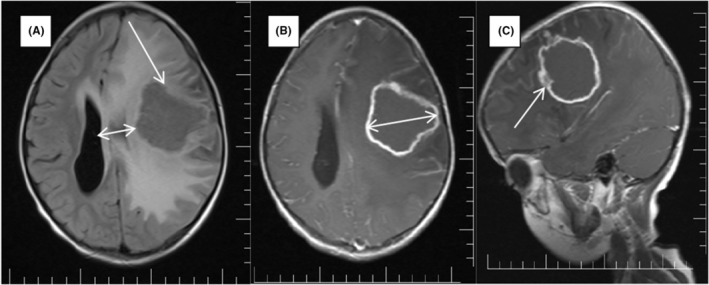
This is an MRI brain that shows a lesion with vasogenic edema in the right frontoparietal region (A), and after IV Gad contrast, it shows lesion with leptomeningeal enhancement of both cerebral hemispheres (B and C).
